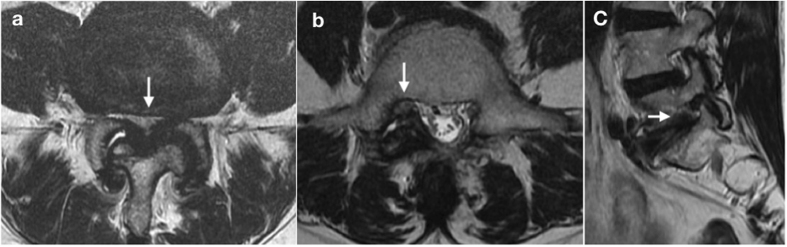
The arrows indicate Schizas’s grade D central stenosis (a) and grade III lateral recess stenosis (b) on axial T2-weighted magnetic resonance images, and grade III foraminal stenosis (c) on a sagittal T2-weighted magnetic resonance image.
